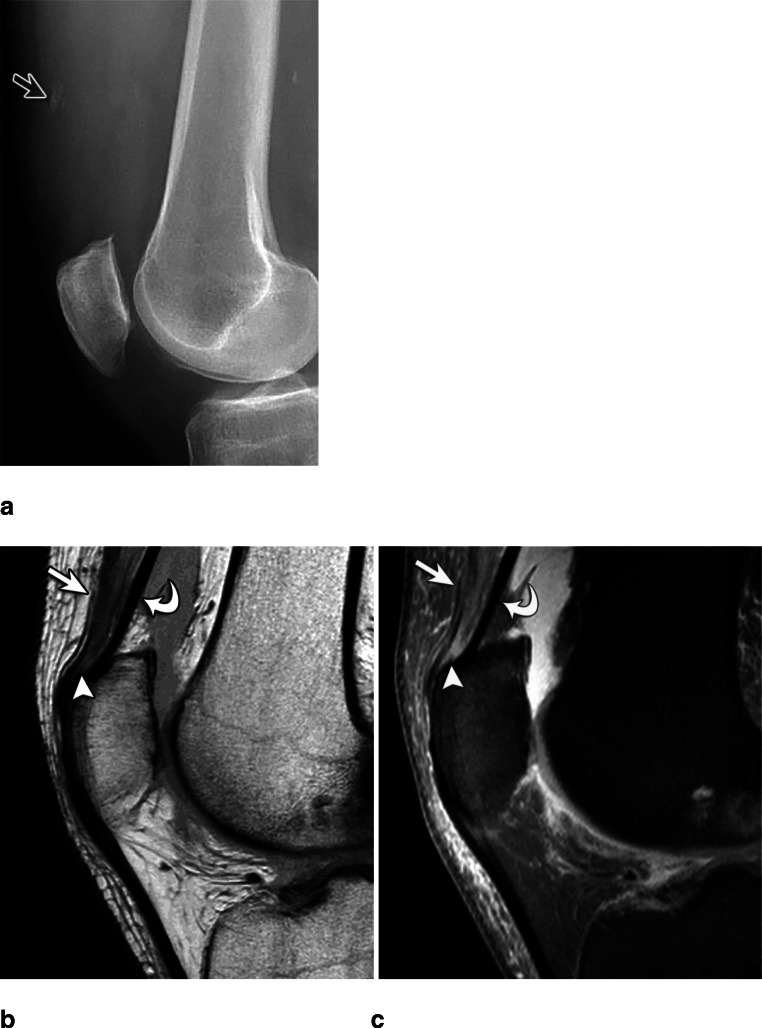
Sixty-year-old male with quadriceps tendon tear and bone avulsion. Radiograph (a) shows a bone avulsion (open arrow). Sagittal intermediate-weighted MR image with (b) and without (c) fat saturation shows complete tears of the rectus femoris (arrow), as well as the combined vastus medialis and lateralis (arrowhead) tendon. The vastus intermedius is normal (curved arrow). The patient was treated conservatively
